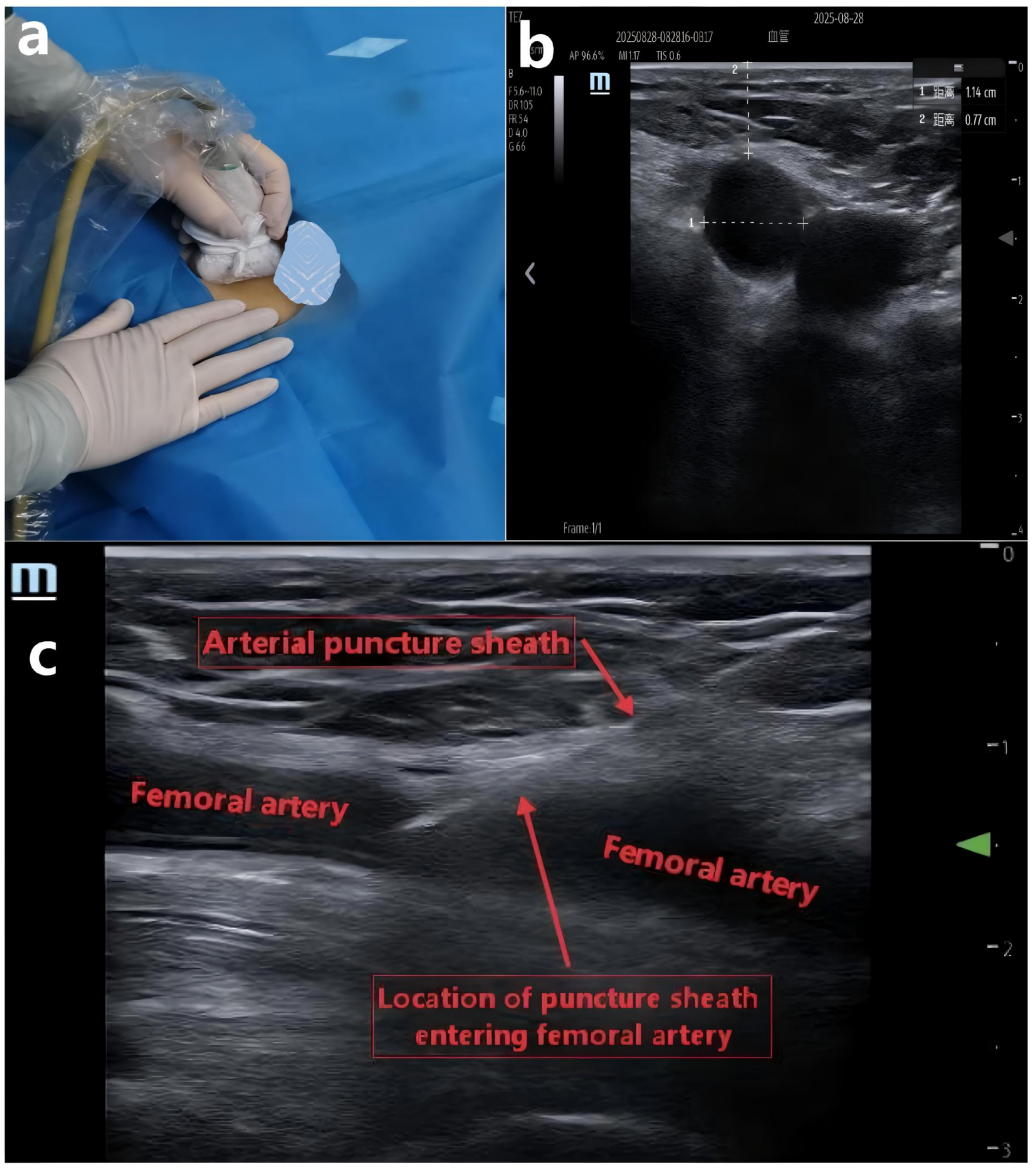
(a) Use ultrasound probe to compress and stop bleeding under ultrasound guidance. (b) Measure the diameter and depth of the femoral artery under ultrasound. (c) Ultrasound showed that the arterial puncture sheath entered the femoral artery.
